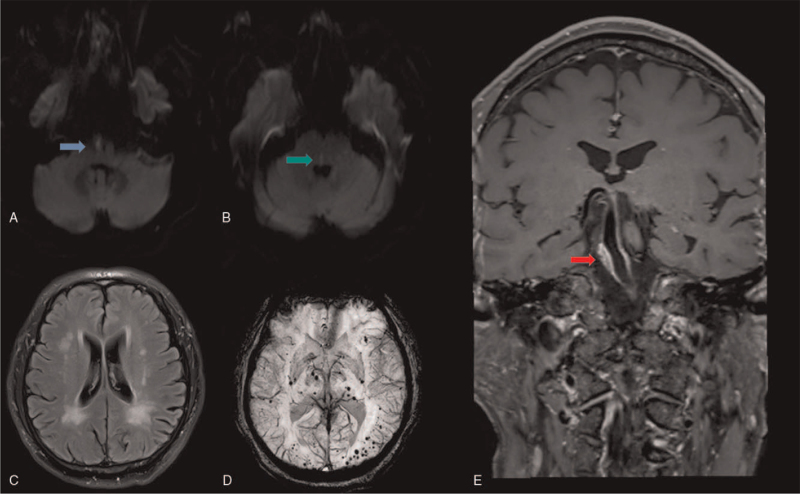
Brain MRI and high-resolution MRI of the arterial wall. (A) DWI suggested high signal in the right medulla oblongata (blue arrow). (B) There was high signal in the right pons (green arrow). (C) FLAIR imaging indicated white matter hyperintensities near the bilateral cerebral ventricles. (D) Cerebral microbleeds on SWI. (E) Localized thickening of the right basilar artery wall with abnormal signals suggesting intramural hematoma on high-resolution MRI (red arrow). DWI = diffusion-weighted imaging, FLAIR = fluid attenuated inversion recovery, SWI = susceptibility weighted imaging.
